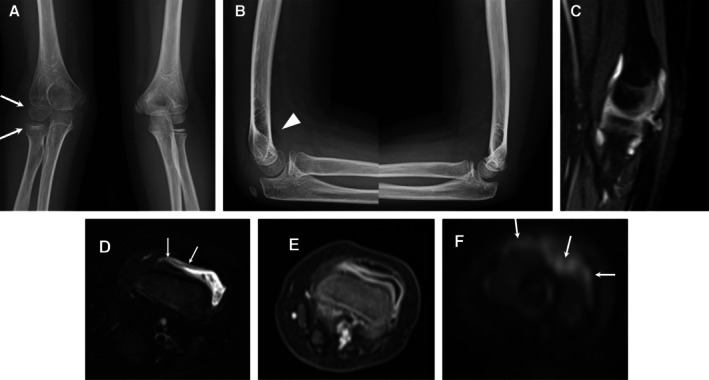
Two cases with ANA‐positive JIA. (A–C) A 7‐year‐old girl with mild pain and restricted extension of the right elbow joint that developed over the past 2 months. Frontal radiographs (A) revealed juxta‐articular osteopenia and epiphyseal overgrowth of the right elbow as compared with the left (arrows). Lateral radiographs (B) showed an elbow fat pad sign (arrowhead) indicating intra‐articular fluid collection. Fat‐suppressed T2‐weighted MR image (C) showed synovial thickening and joint effusion. (D–F) A 2‐year‐old girl with a 2‐month history of left knee pain, followed by joint contracture. Fat‐suppressed T2‐weighted image (D) showed high‐signal fluid accumulation and slightly lower‐signal synovial thickening (arrows). (E) Dynamic contrast‐enhanced MRI revealed early enhancement of the thickened synovium. (F) Diffusion‐weighted image showed restricted diffusion in the synovium (arrows).
